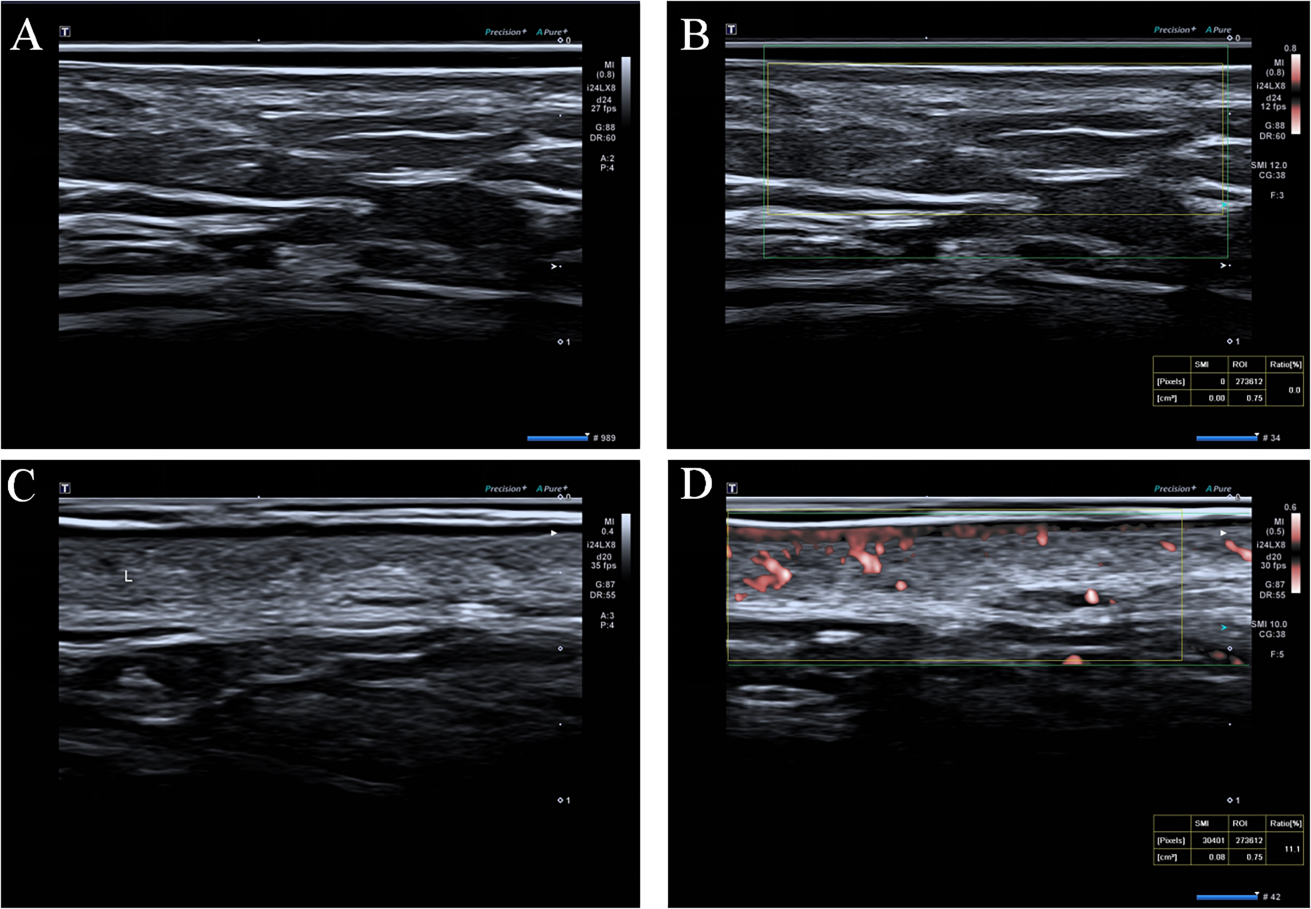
(A) Gray-scale ultrasound images of the control group; (B) VI measurement image of microvascular blood flow for the control group; (C) Gray-scale ultrasound images of the LVD group; (D) VI measurement image of microvascular blood flow for the LVD group.
